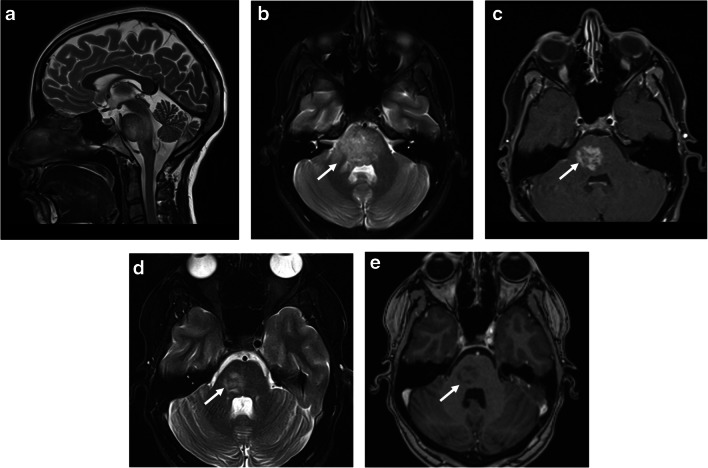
Brainstem involvement in a 17-year-old girl with myelin oligodendrocyte glycoprotein antibody-associated disease, who presented acutely with diplopia, ataxia, and left side hemiparesis. a Mid-sagittal T2-weighted, (b) axial fat-suppressed T2-weighted, and c axial fat-suppressed contrast-enhanced T1-weighted magnetic resonance (MR) images show an ill-defined lesion infiltrating the pons and right middle cerebellar peduncle (*arrow* in b) with mass effect on the adjacent fourth ventricle, associated with abnormal enhancement (*arrow* in c). d Axial fat-suppressed T2-weighted and e axial contrast-enhanced T1-weighted MR images obtained at 2-month follow-up show an interval decrease in size of the lesion (*arrow* in d), with resolution of enhancement (*arrow* in e)
